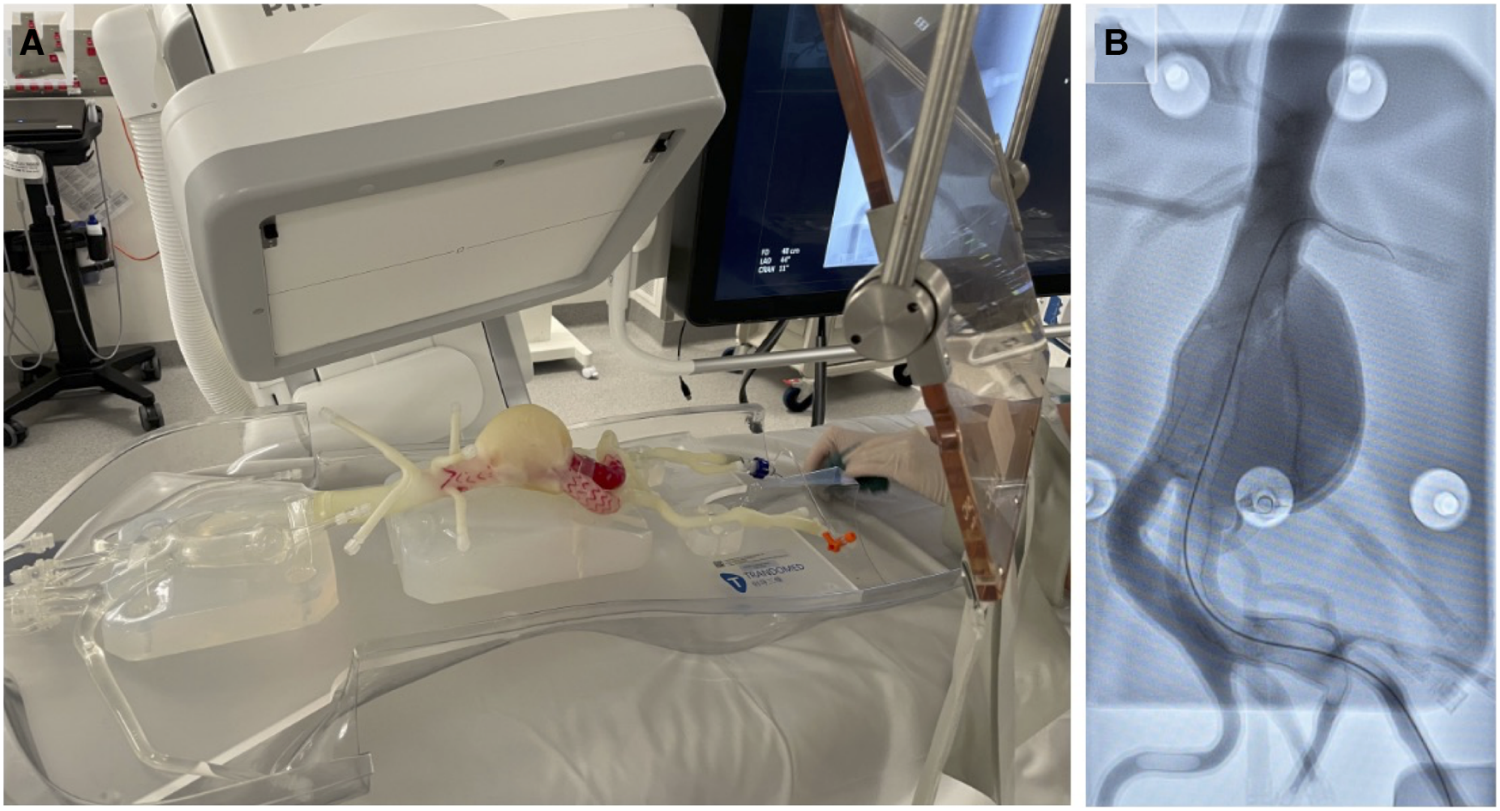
Example application of M3DP abdominal aortic aneurism (AAA) model for surgical training. (A) Perfused AAA model situated under x-Ray C-Arm and (B) representative x-Ray image showing contrast-enhanced model and surgical guide wire.
